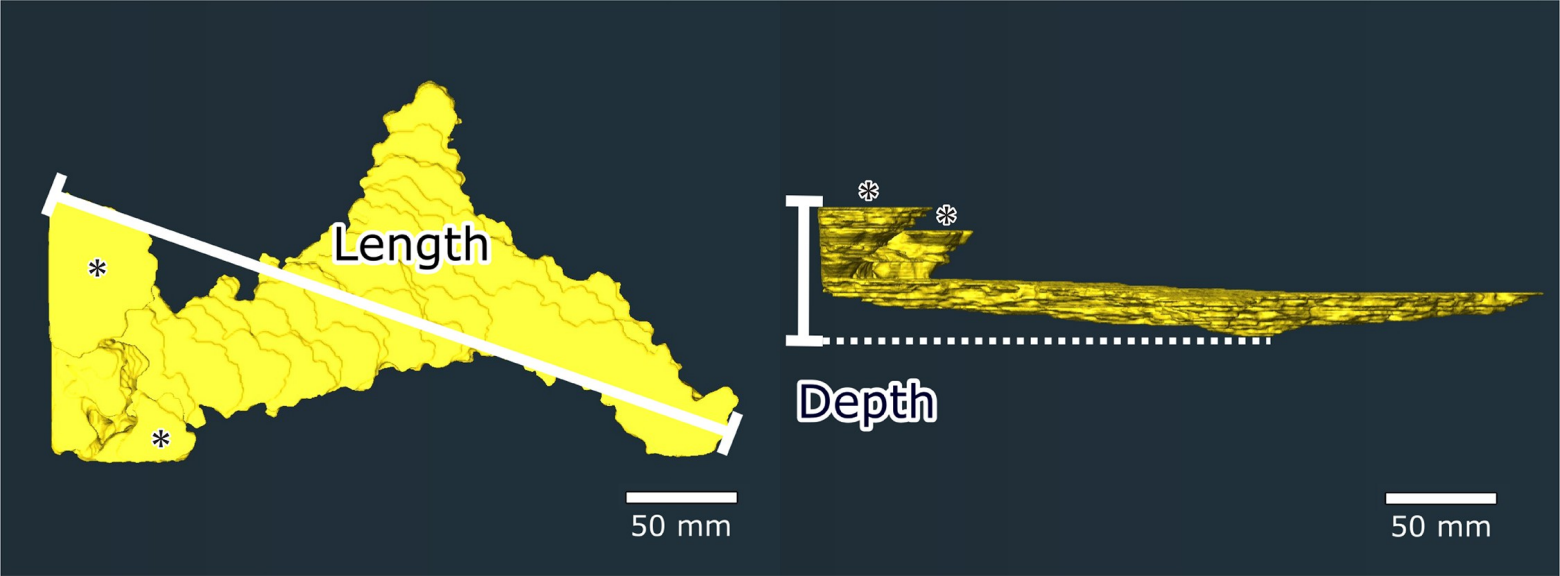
Measurements of burrow length and depth from computed tomography images. The left-hand and right-hand panels show top and side views, respectively. The asterisks show the burrow apertures.
